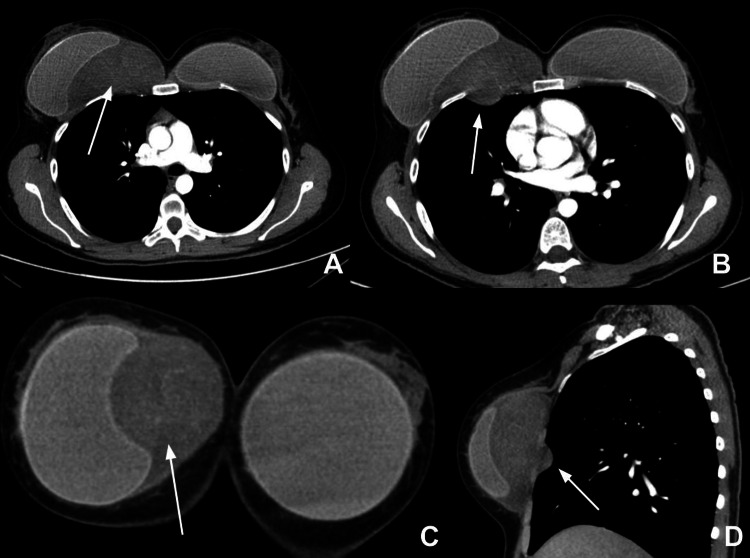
Chest computed tomography with contrast: Axial sections (A and B) show a round hypodense lesion with linear contrast enhancement with mild invagination towards the ipsilateral pulmonary parenchyma (arrows). Coronal reconstruction (C) shows displacement and deformity of the right breast prosthesis without infiltration (arrow). Sagittal reconstruction (D) confirms the posterior location of the lesion in relation to the implant and pulmonary invagination without capsular or adjacent tissue compromise (arrow).
